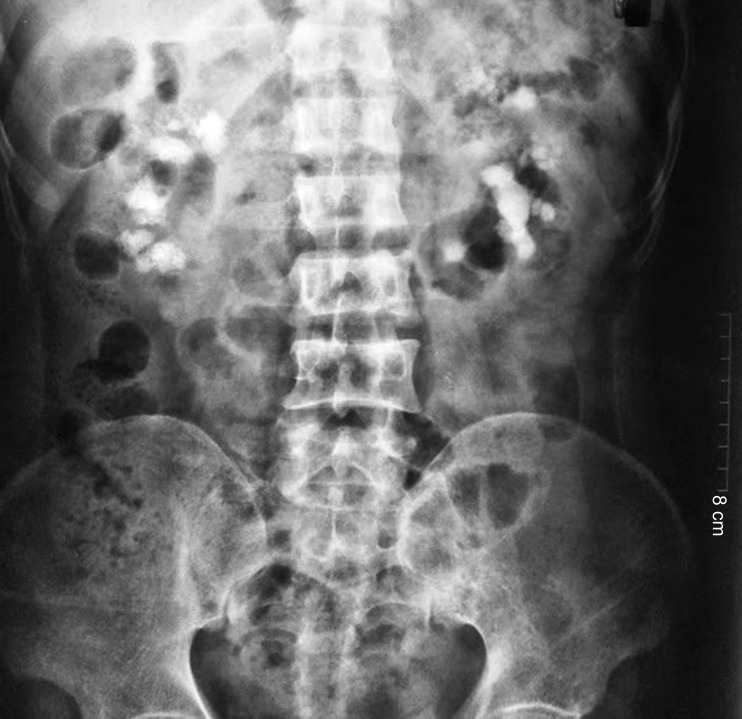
Straight X-ray abdomen showing bilateral staghorn renal calculus without any hydronephrotic changes.
